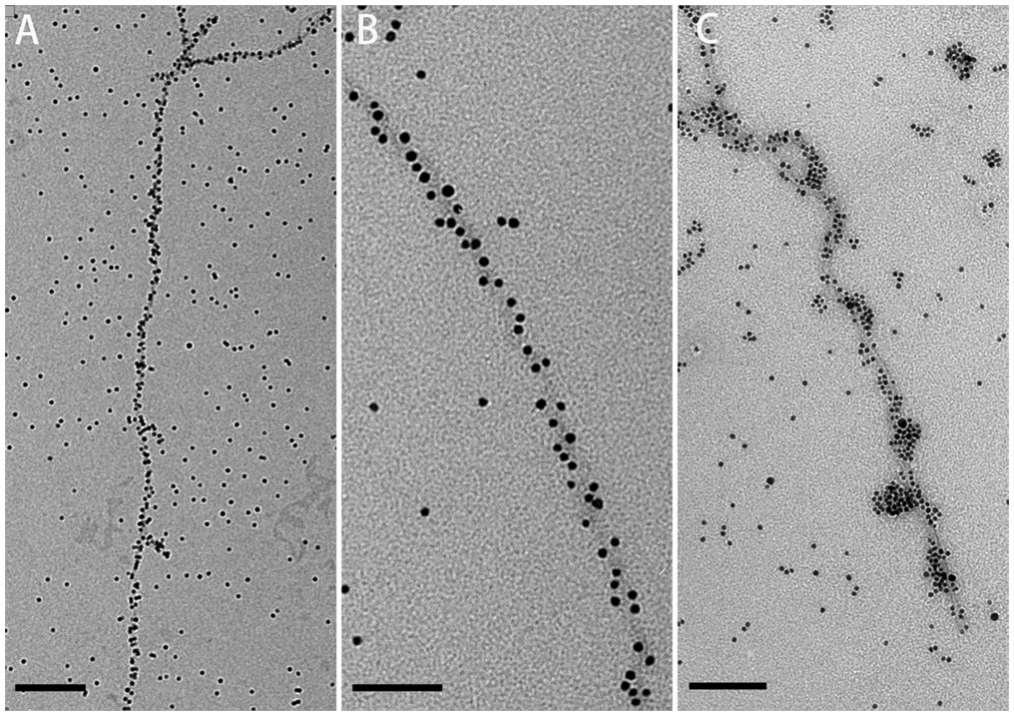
TEM images of representative gold-labelled fibrils. A & B) RiAFP-m9 fibrils labelled with 5 nm AuNPs. C) SBAFP-CT fibrils labelled with 5 nm AuNPs. Scale bar: A) 200 nm; B) 60 nm: C) 100 nm.
